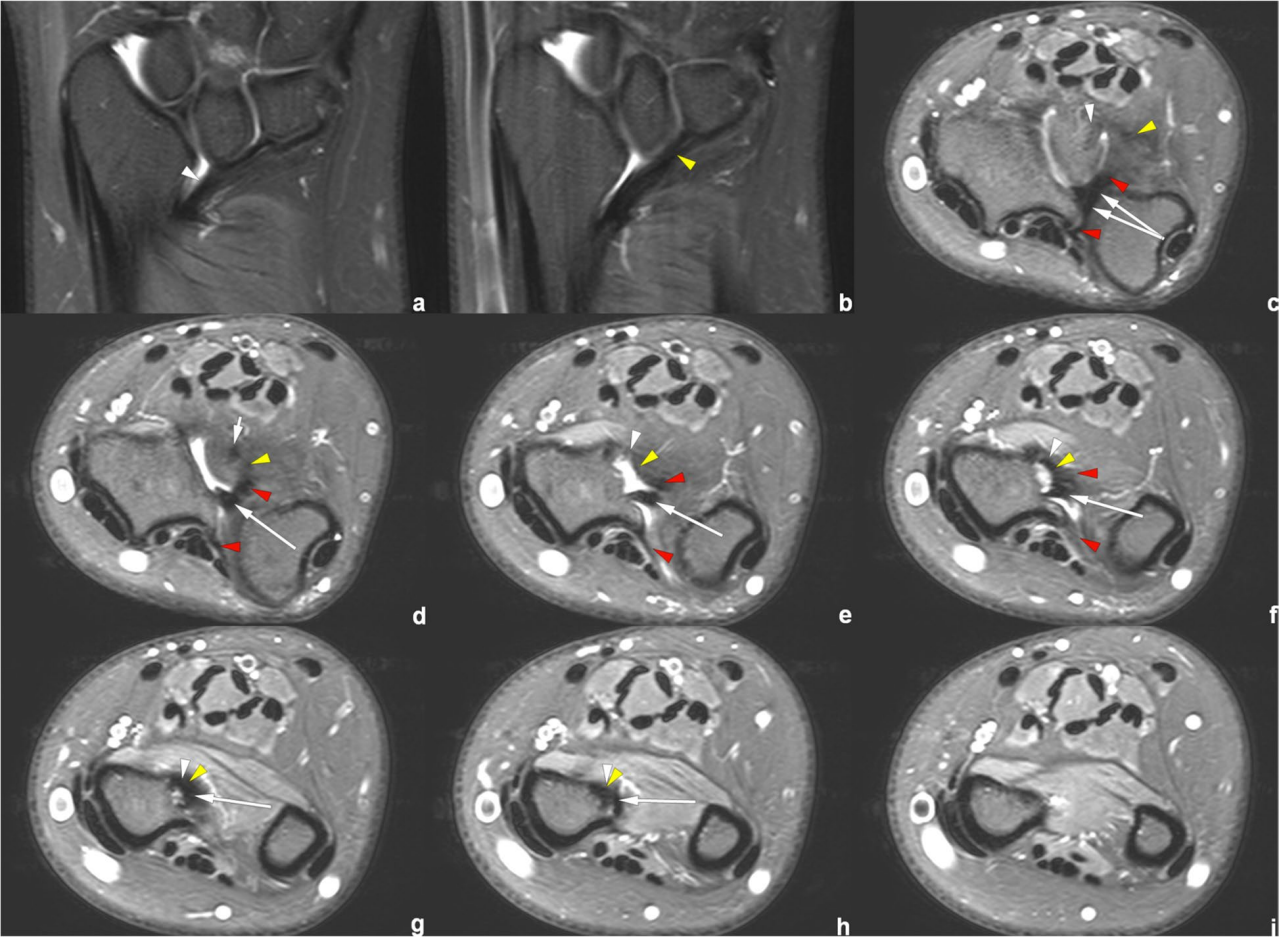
Left wrist MRI of the patient No. 0621. a–b Coronal PDWI-FS images show the VL (white arrowhead) and RTL (yellow arrowhead). c–i Axial PDWI-FS images show the CD (white arrow), volar and distal radioulnar ligament (red arrowhead), VL (white arrowhead), and RTL (yellow arrowhead)
